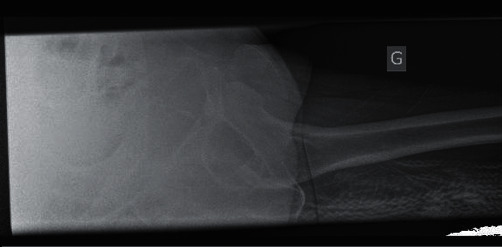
Axial view radiograph confirming the anterior dislocation.
